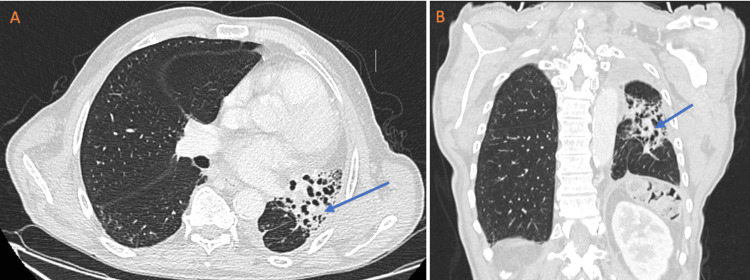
Thoracic computed tomography (CT) scan A: transverse section; B: coronal section Follow-up imaging of the chest revealed stable post-treatment changes, including expected fibrotic alterations (arrow), a reduction in left lung volume, and a slight left pleural effusion.
